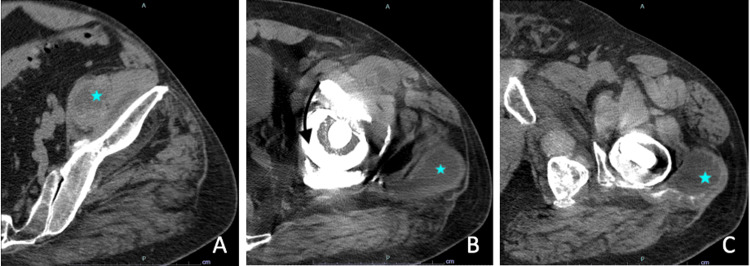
Successive axial cuts of CT scan left hip without contrast (A) Psoas fluid collection (blue star); (B) acetabular protrusio and medial wall loss (black arrow), along with soft tissue fluid collections around the iliopsoas and a large collection around the greater trochanter to the level of the fascia (blue star); (C) fluid collection around the greater trochanter (blue star).
